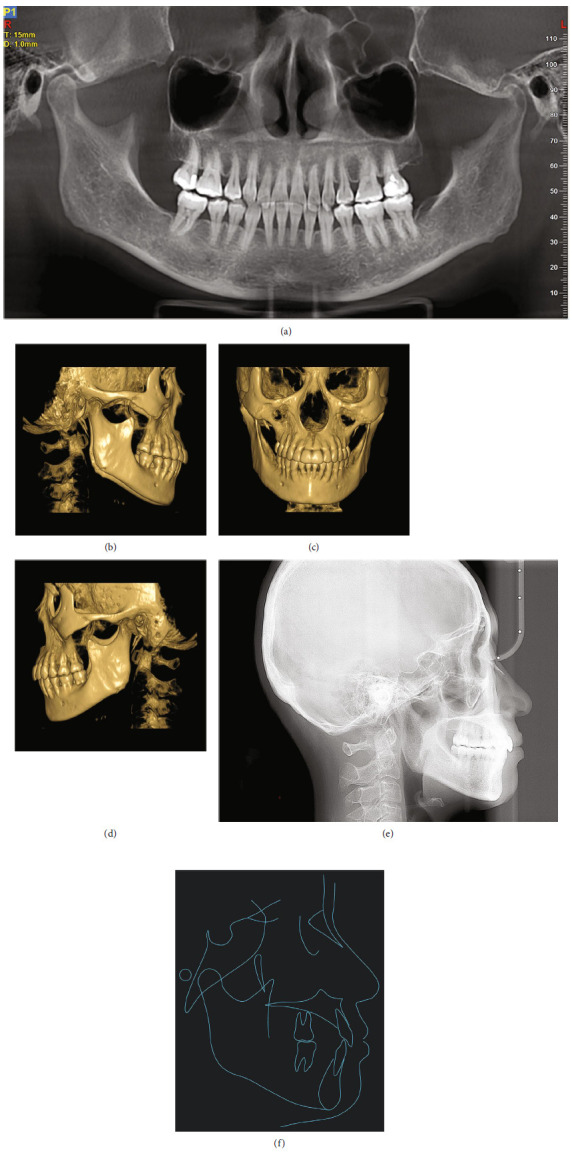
Posttreatment radiographs. (a) Panoramic radiograph sectioned from CBCT. (b–d) Three-dimensional reconstruction of CBCT. (e) Lateral cephalogram. (f) Lateral cephalometric tracing.
